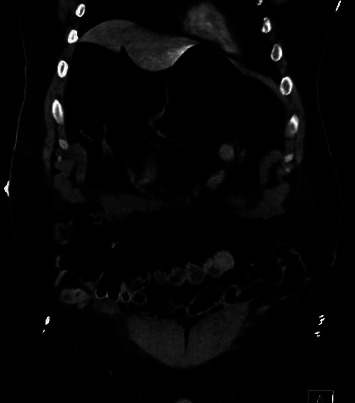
Abdominal CT. Absence of the rectus muscles with resultant bowel herniation.
